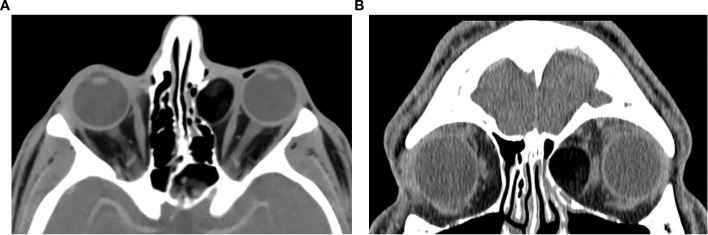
Axial (a) and coronal (b) post-contrast CT images of the orbits in a 26-year-old male patient reveal a well-defined, mostly fatty, extraconal lesion located nasally, medial to the medial rectus muscle in the left orbit. The lesion demonstrates predominantly fat attenuation (approximately –105 HU), with slightly denser internal components and peripheral thin rim enhancement. In this location, the appearance favors a dermoid cyst, whose lipid-rich contents produce fat attenuation, overall consistent with a benign cystic lesion. The mass exerts a moderate mass effect on the left medial rectus muscle with slight globe displacement and causes thinning and lateral bowing of the adjacent left lamina papyracea, suggestive of a chronic benign process. The diagnosis was confirmed on excision.
